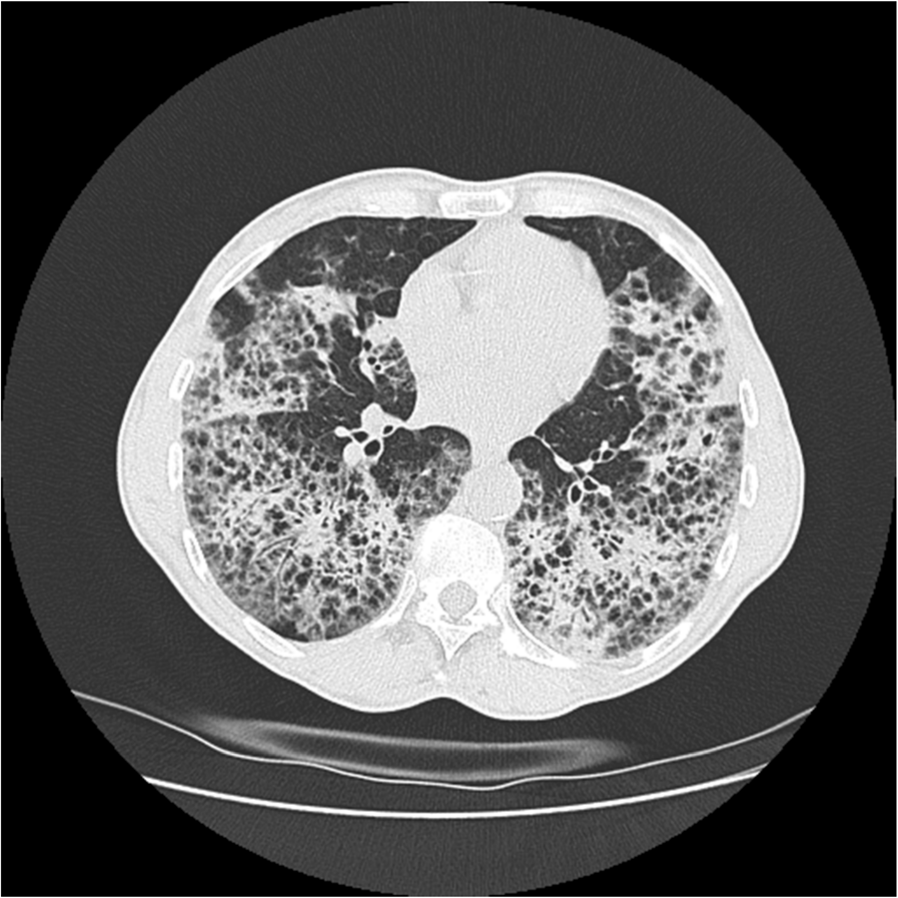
Computed tomography scan of the thorax with axial view demonstrating of reticular interstitial opacities with plenty of thick mucus, and thickening of interlobular septa
